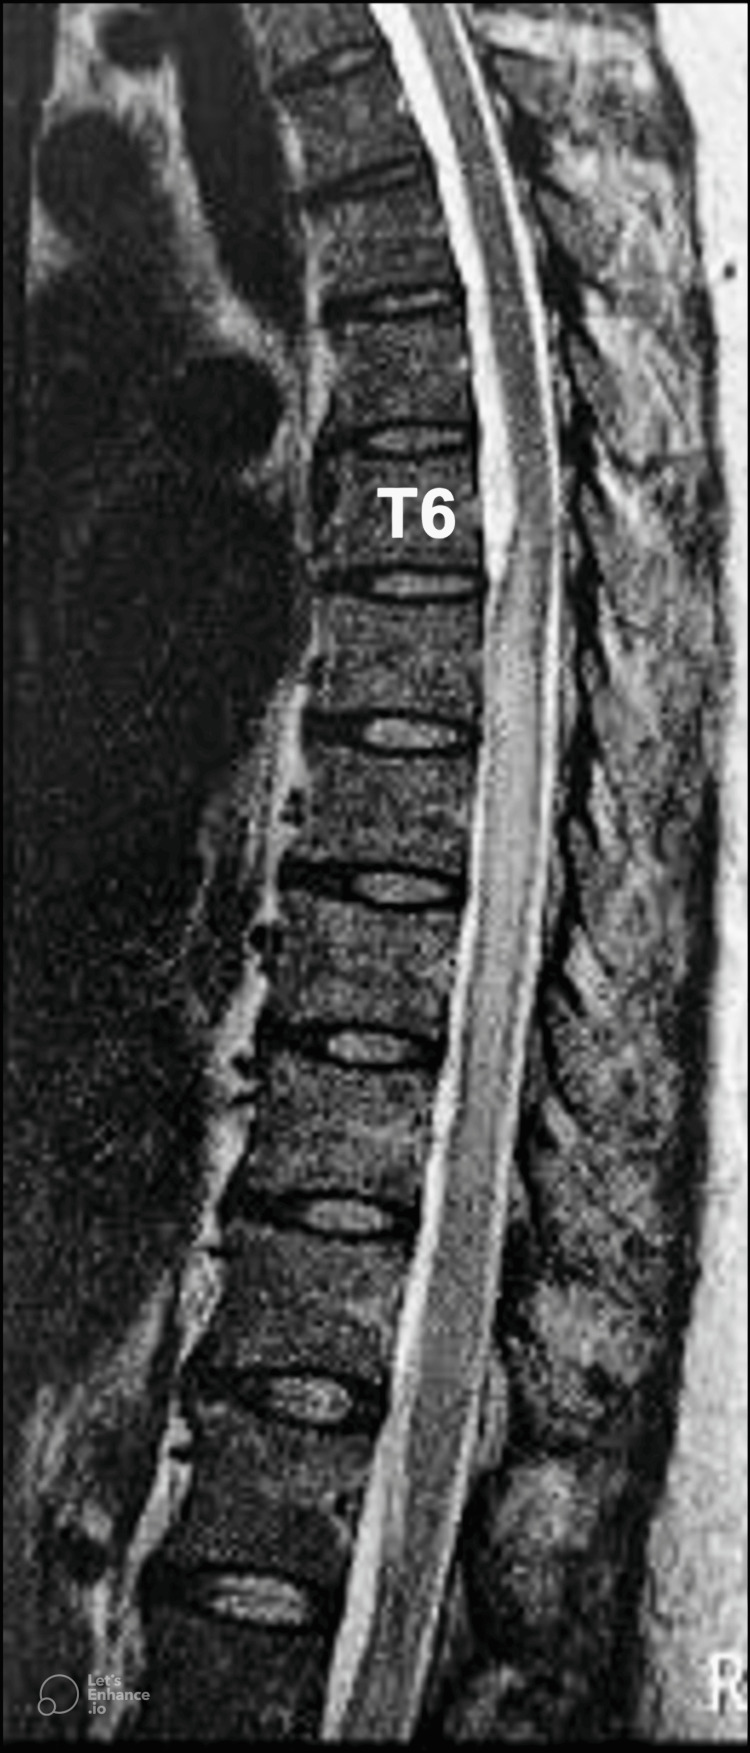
Sagittal T2-weighted MRI of the thoracolumbar spine three months after spinal anesthesia indicating edema of the spinal tissue and a cystic mass at the T5 level, causing mild compression of the dorsal column.
